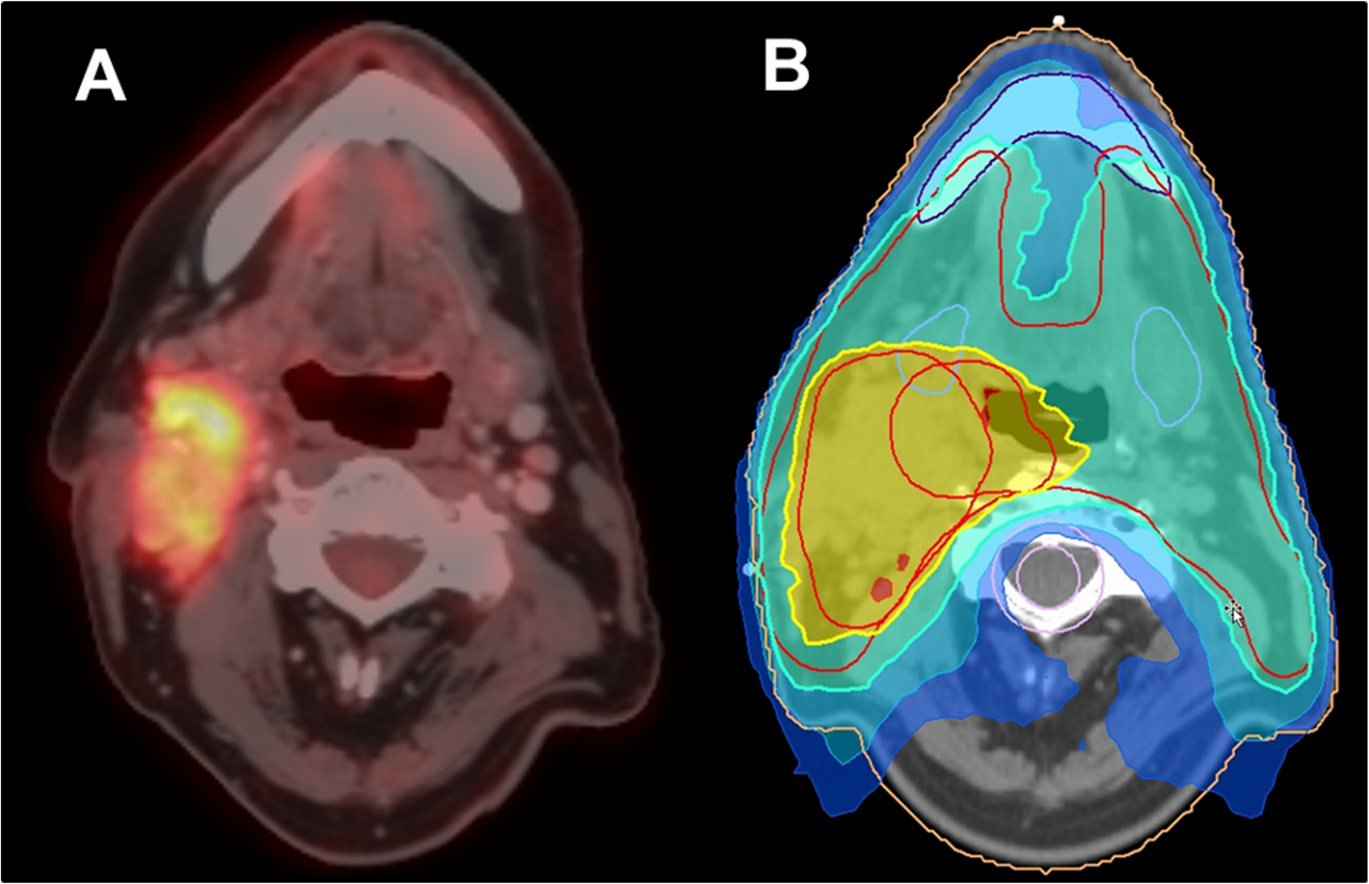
Patient with HPV-positive squamous cell carcinoma of the right tonsil (T1 cN3 M0), who underwent ^18^F-FDG-PET/CT for staging prior to radiotherapy and subsequent inclusion of PET-positive tumor masses and lymph nodes in radiotherapy planning
